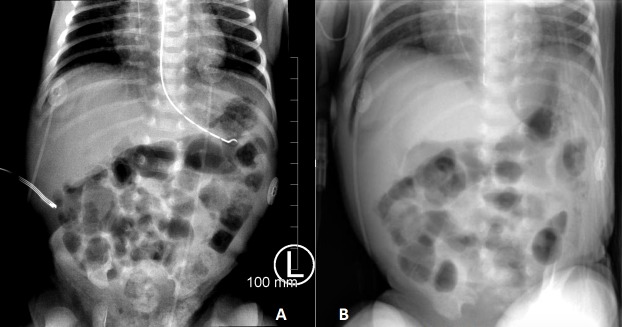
Figure 1: A) Initial plain abdominal radiograph demonstrating pneumatosis intestinalis. B) Follow-up plain abdominal radiograph demonstrating intra-peritoneal, extra-luminal gas.
